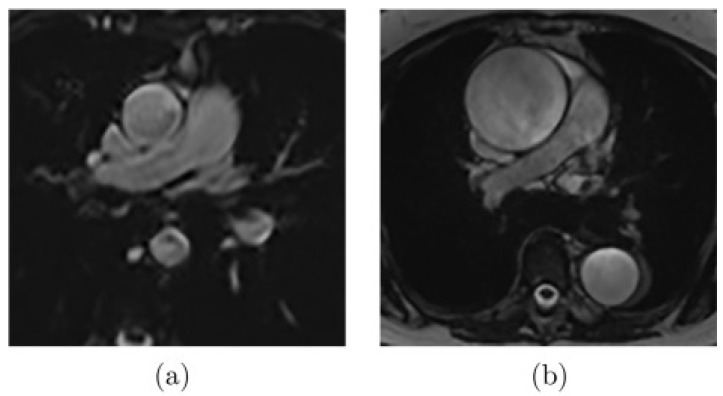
Examples of MR images where (a) the aortic wall is not well-defined due to the influence of the proximity of other structures or (b) the aorta is highly dilated [20].
